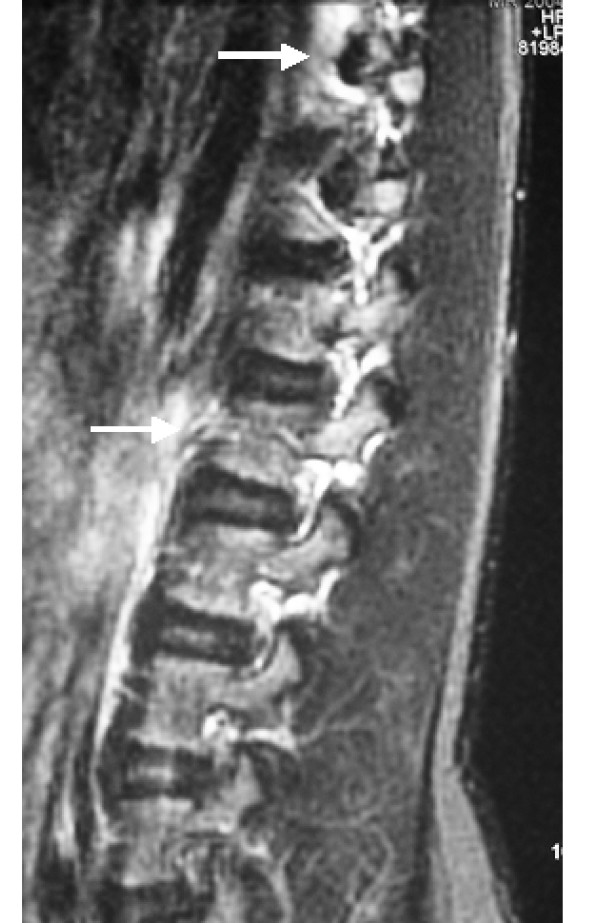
Magnetic resonance imaging of the lower thoracic spine with sagittal T2 fast spin echo sequences showing the ossified anterior longitudinal ligament with subsequent anterior vertebral hyperostosis and bridging (arrows). In addition there was involvement of the posterior longitudinal ligament. Peripheral sclerotic borders associated with anterior end plate irregularities have outlined the overall vertebral bodies.
